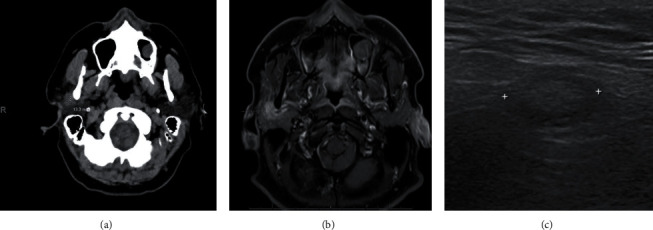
Imaging of the right parotid gland. (a) CT with contrast demonstrating increased soft tissue density. (b) MRI demonstrating mass-like enhancement. (c) Ultrasound demonstrating a poorly defined nodule.
